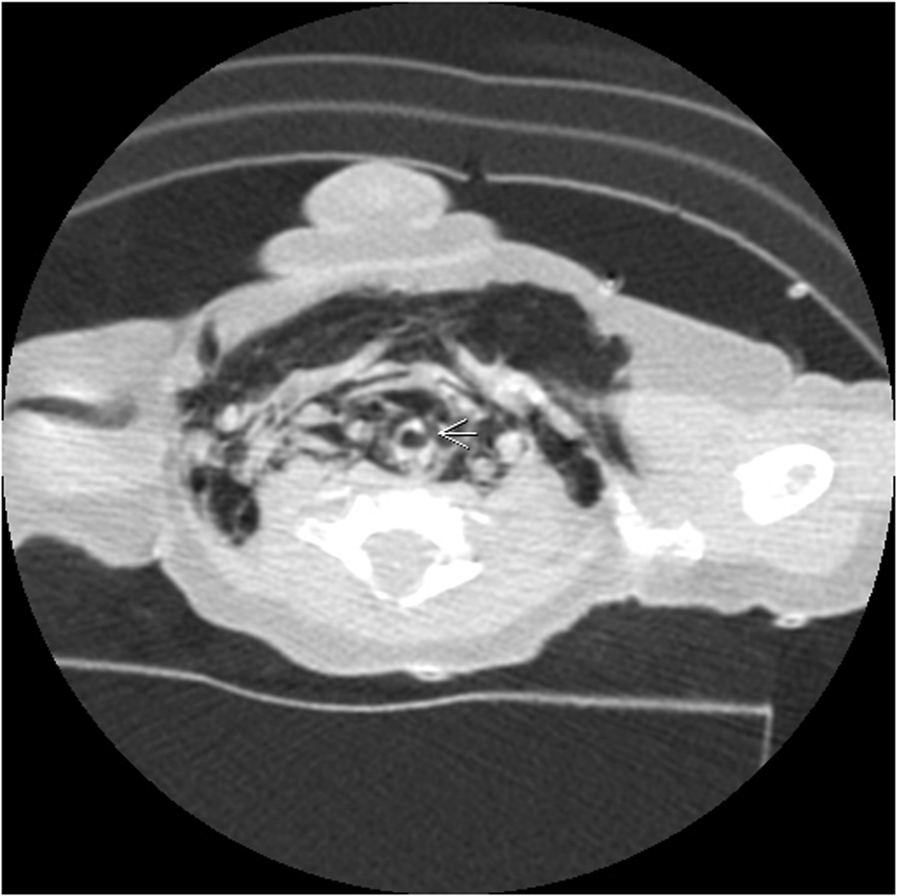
Computed tomography of the neck. Arrow pointing to a defect involving the left lateral wall of upper trachea with associated extensive pneumomediastinum and subcutaneous emphysema
